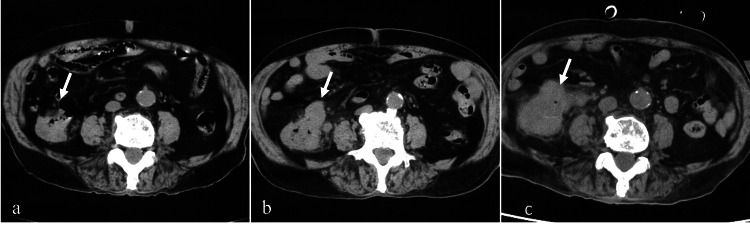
Abdominal computed tomography of case 2. Abdominal computed tomography scans at various time points are as follows: at the time of diagnosis (a), six months (b), and 18 months (c). The tumor from the terminal ileum to the proximal ascending colon shows progressive enlargement over time (arrows).
